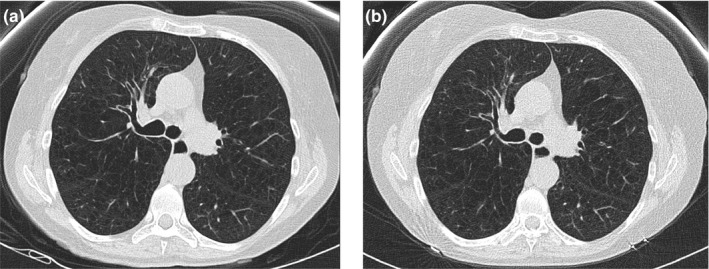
Axial images of the smallest sized patient who received annual lung cancer screening CT examinations scanned with (a) FTC the first year and (b) TCM the second year. FTC, fixed tube current; TCM, tube current modulation.
